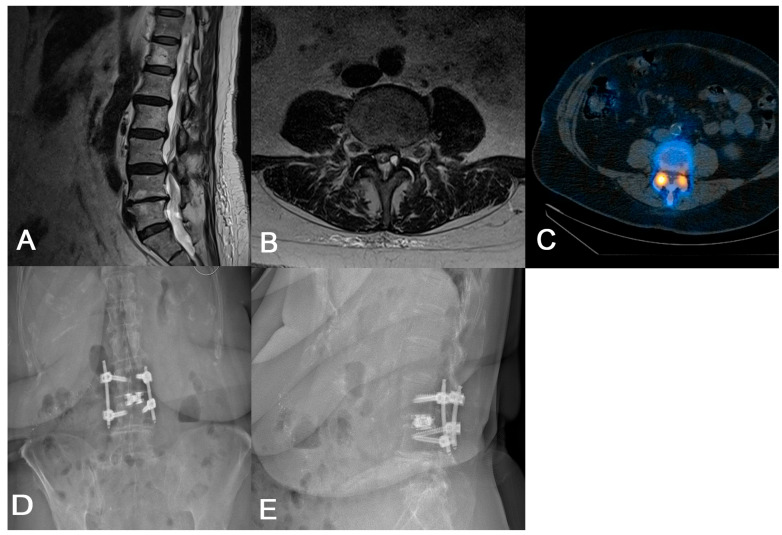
(A) Preoperative sagittal lumbar MRI demonstrating L3/4 spondylolisthesis. (B) Preoperative axial lumbar MRI showing a cyst on the L3/4 facet in addition to nerve root compression. (C) Preoperative axial CT SPECT showing increased radiotracer uptake in the bilateral L3/4 facet joints. (D,E) Postoperative AP and lateral lumbar radiographs demonstrating appropriate implant placement.
